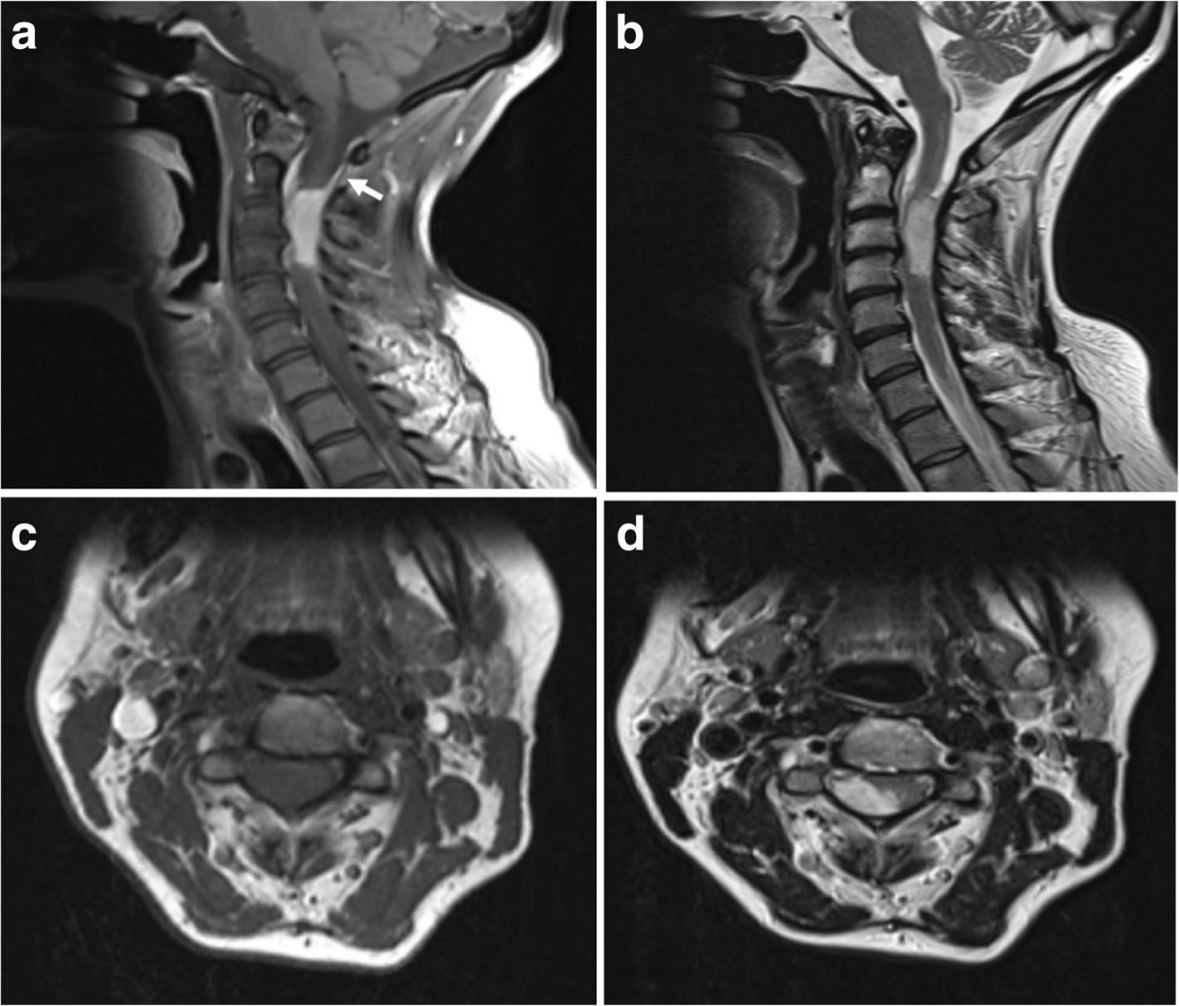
Dural glioblastoma metastasis. a Post-contrast sagittal T1-weighted MR image showing an avidly enhancing intra-dural, extra-medullary lesion within the upper posterior cervical spine. An enhancing dural tail is seen superiorly (white arrow). b T2-weighted sagittal images of the same lesion. c, d Axial T1 and T2-weighted images show the posterior intra-dural and extra-medullary location of the lesion which is compressing the cord to the left
